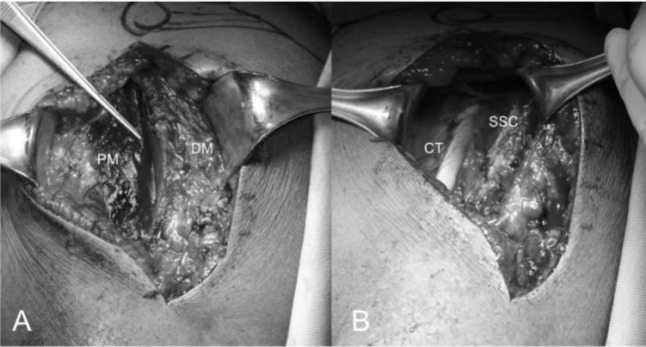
A–B: Deltopectoral interval with the cephalic vein along the edge of the pectoralis major (A), conjoint tendon and subscapularis tendon. PM: pectorali major; DM: deltoid muscle; CT: conjoint tendon; SSC: subscapularis
